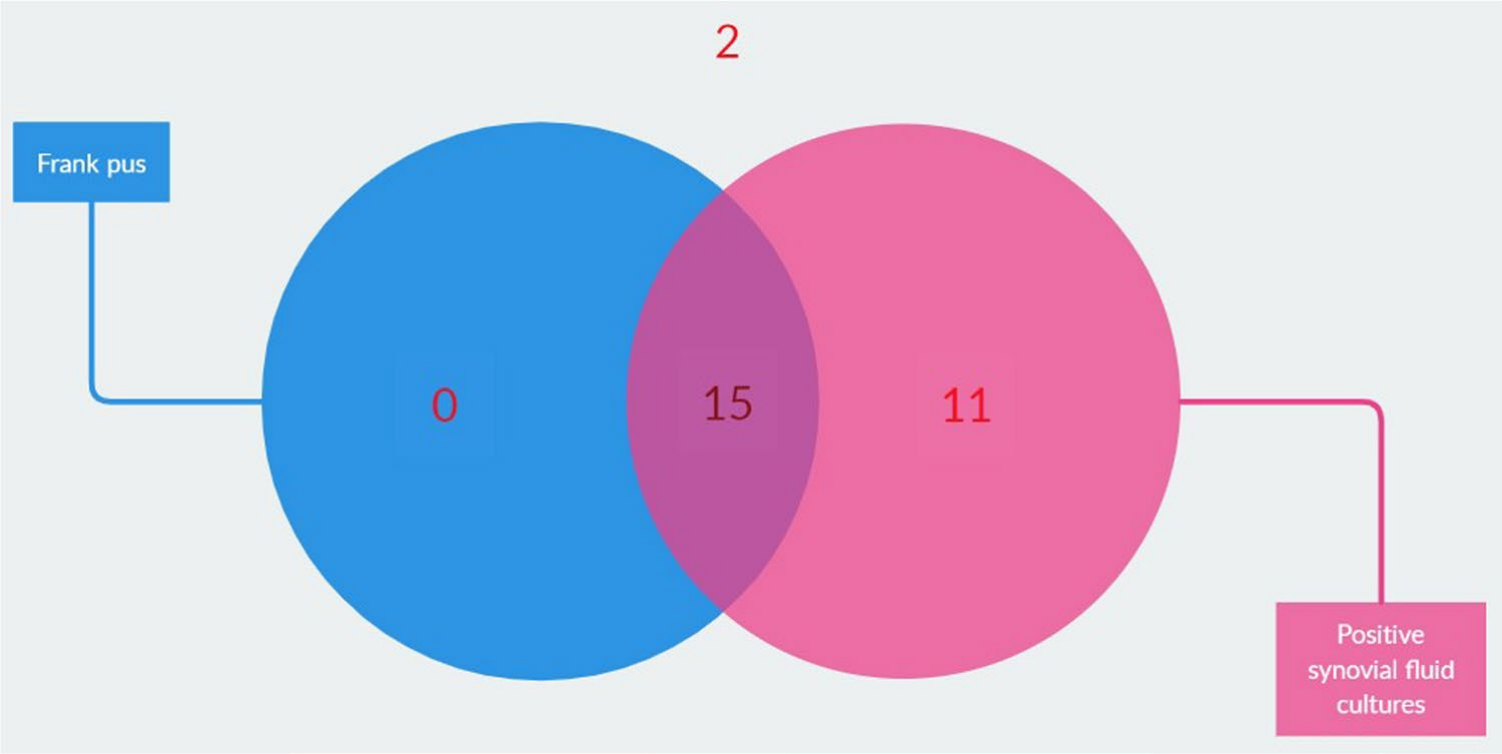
Distribution of septic arthritis patients with frank pus or positive synovial fluid cultures. The two patients without positive synovial fluid cultures or frank pus had radiographical indications and positive cultures from surgical samples
